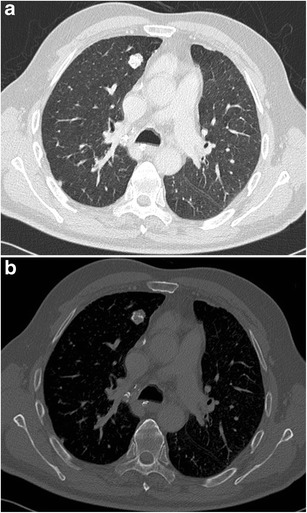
Incidental finding in a 75-year-old man. Axial CT-image in lung window setting a shows a well delineated somewhat lobulated nodule in the right upper lobe. In bone window b the chunky popcorn calcifications can easily be appreciated. Findings are consistent with a hamartoma
